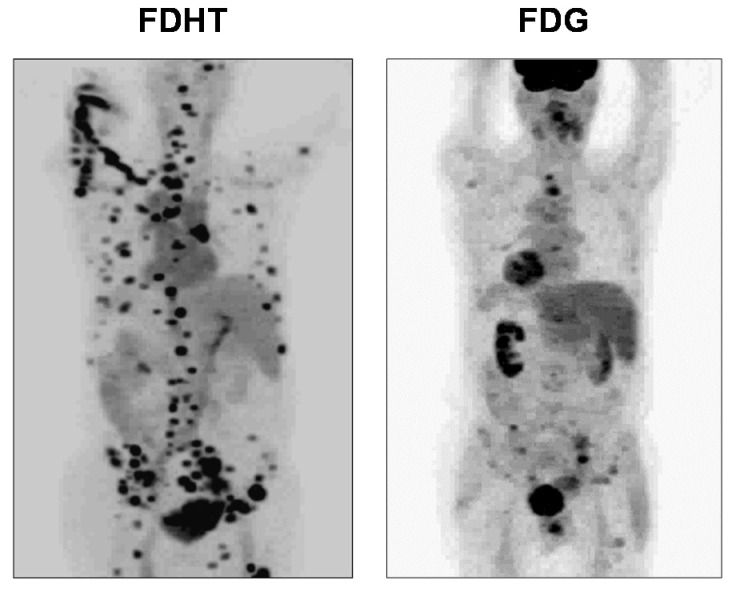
FDHT-PET and FDG-PET image of prostate cancer in an individual with FDHT-avid disease. (Images provided by Dr. Steven Larson, Memorial Sloan Kettering Cancer Center.)
